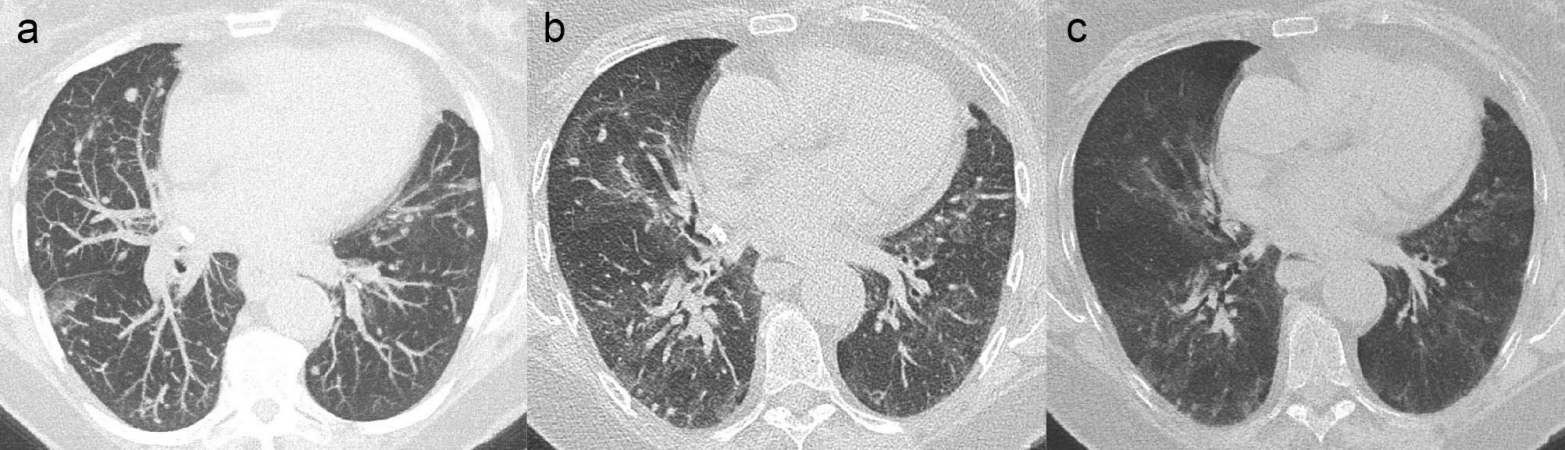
DIPNECH in an oncologic female patient. Diffuse idiopathic pulmonary neuroendocrine cell hyperplasia (DIPNECH) in a 63 y.o. woman who underwent gastric resection due to gastric adenocarcinoma. Axial computed tomography (CT) maximum intensity projection (MIP) image (a), expiratory low dose image (b) with minimum intensity projection (MinIP) reconstruction (c) showing well-defined bilateral nodules of various size and diffuse inhomogeneous density of lung parenchyma due to air trapping, better depicted in the expiratory images
